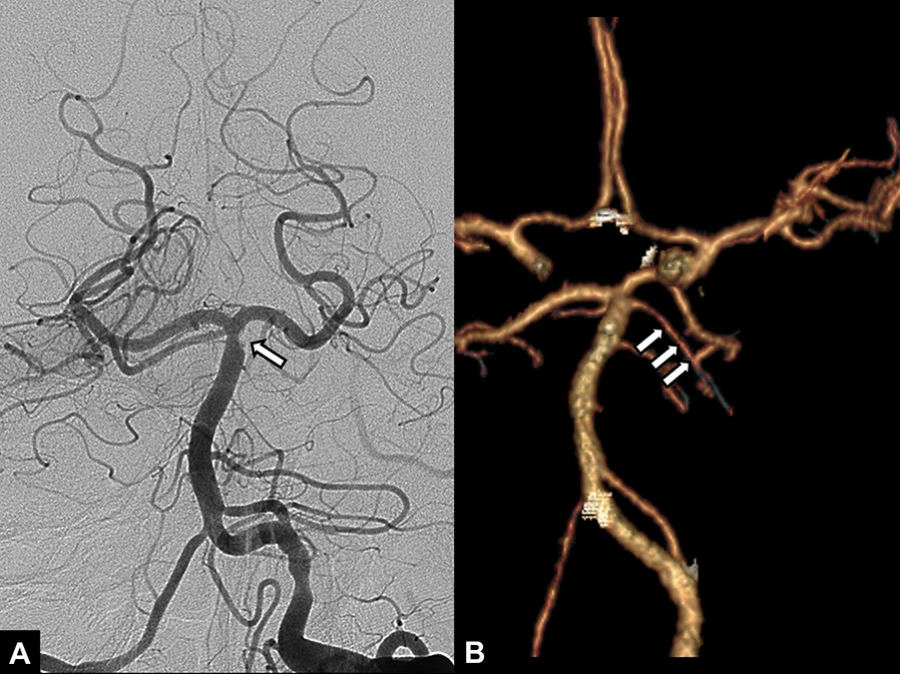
A 50-year-old male patient with acute stroke due to basilar artery occlusion. (A) Final angiogram obtained after stent-retriever thrombectomy shows an occlusion (arrow) at the ostium of the left superior cerebellar artery. (B) Follow-up CT angiography obtained 40 h after thrombectomy shows spontaneous recanalization of the previously occluded left superior cerebellar artery (arrows).
